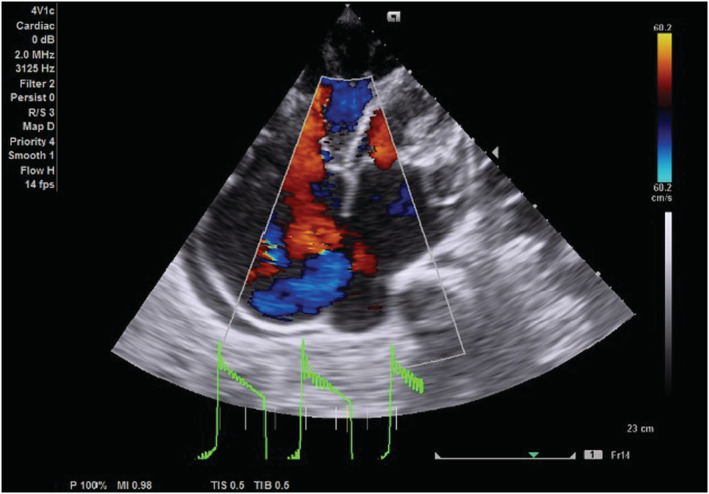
Epical 4 chamber color Doppler echocardiogram showing a large atrial septal defect with bidirectional flow.
